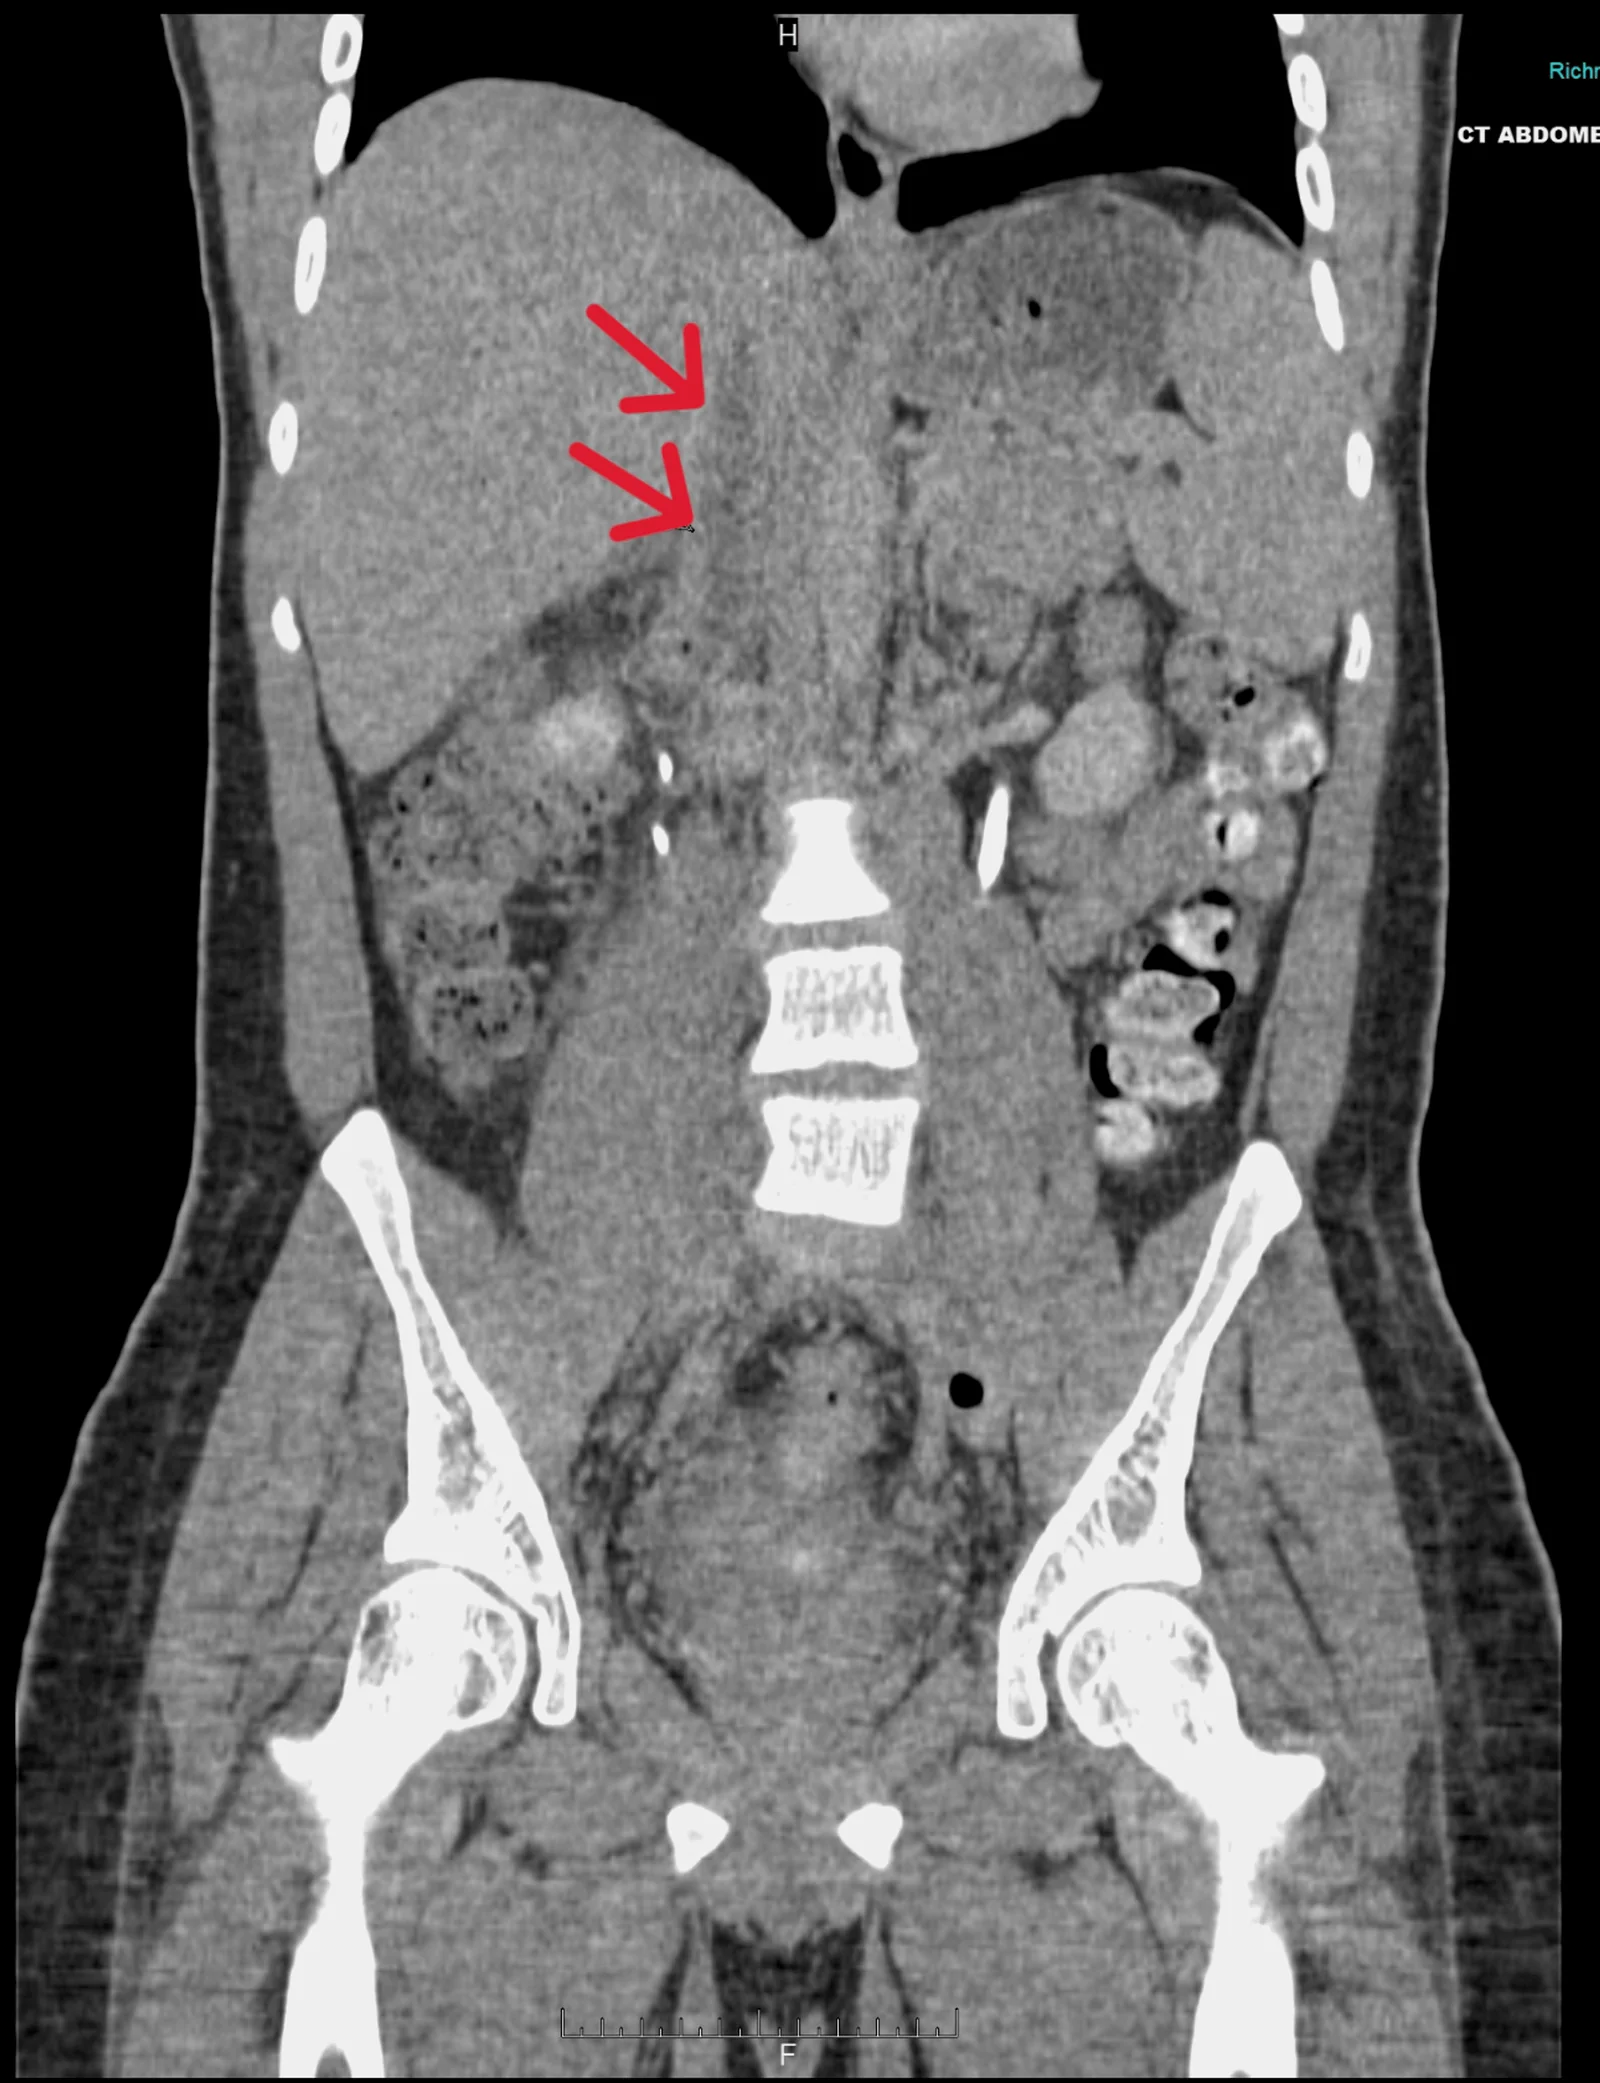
Coronal computed tomography angiography demonstrating extension into the intrahepatic inferior vena cava and right renal vein (red arrows)
